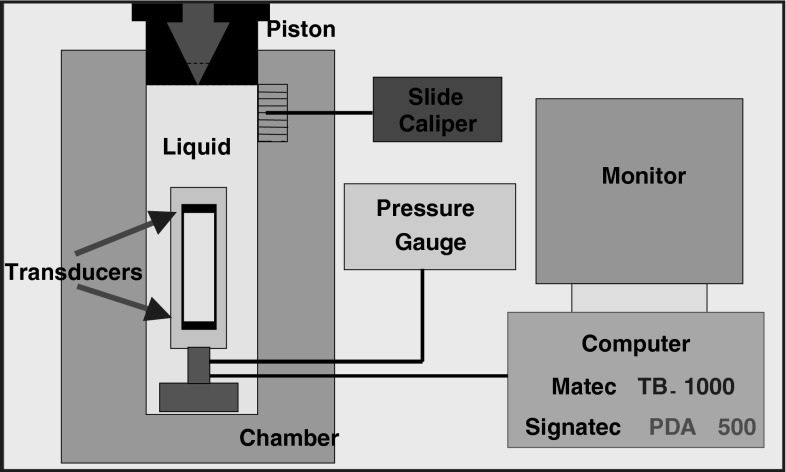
Experimental setup for measuring longitudinal ultrasonic wave velocity in liquids at high pressure
